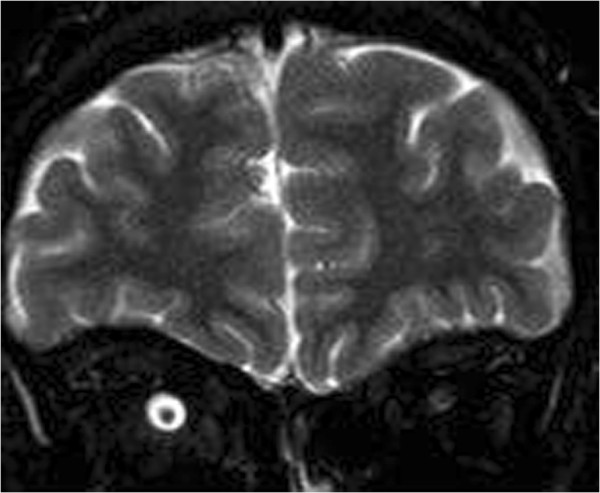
Compression of the optic nerve, right side, magnetic resonance imaging.
